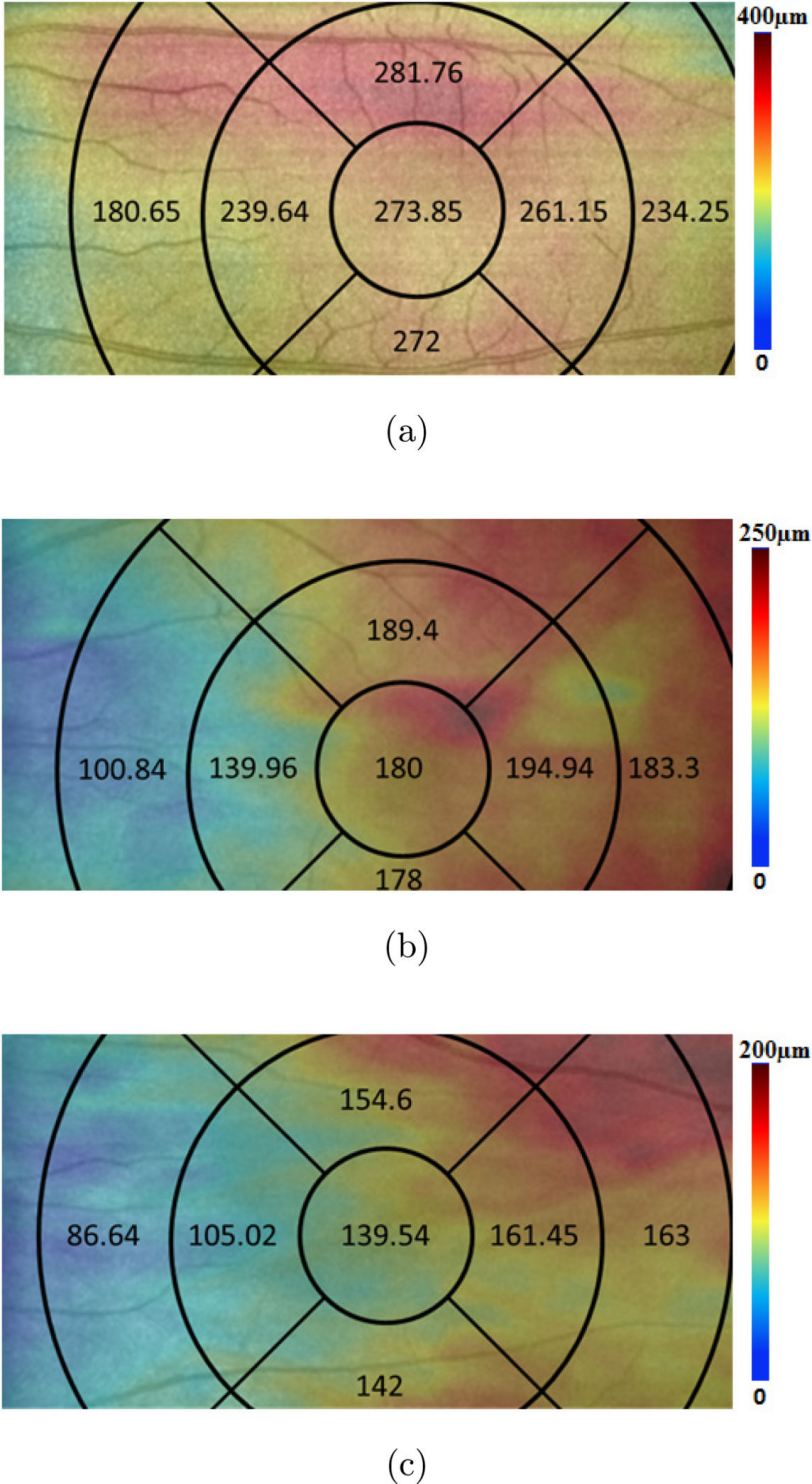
The mean CT distribution in different superior, inferior, nasal, and temporal quadrants under conditions of low (a), moderate, (b) and high myopia (c). Data are presented as the mean value.
